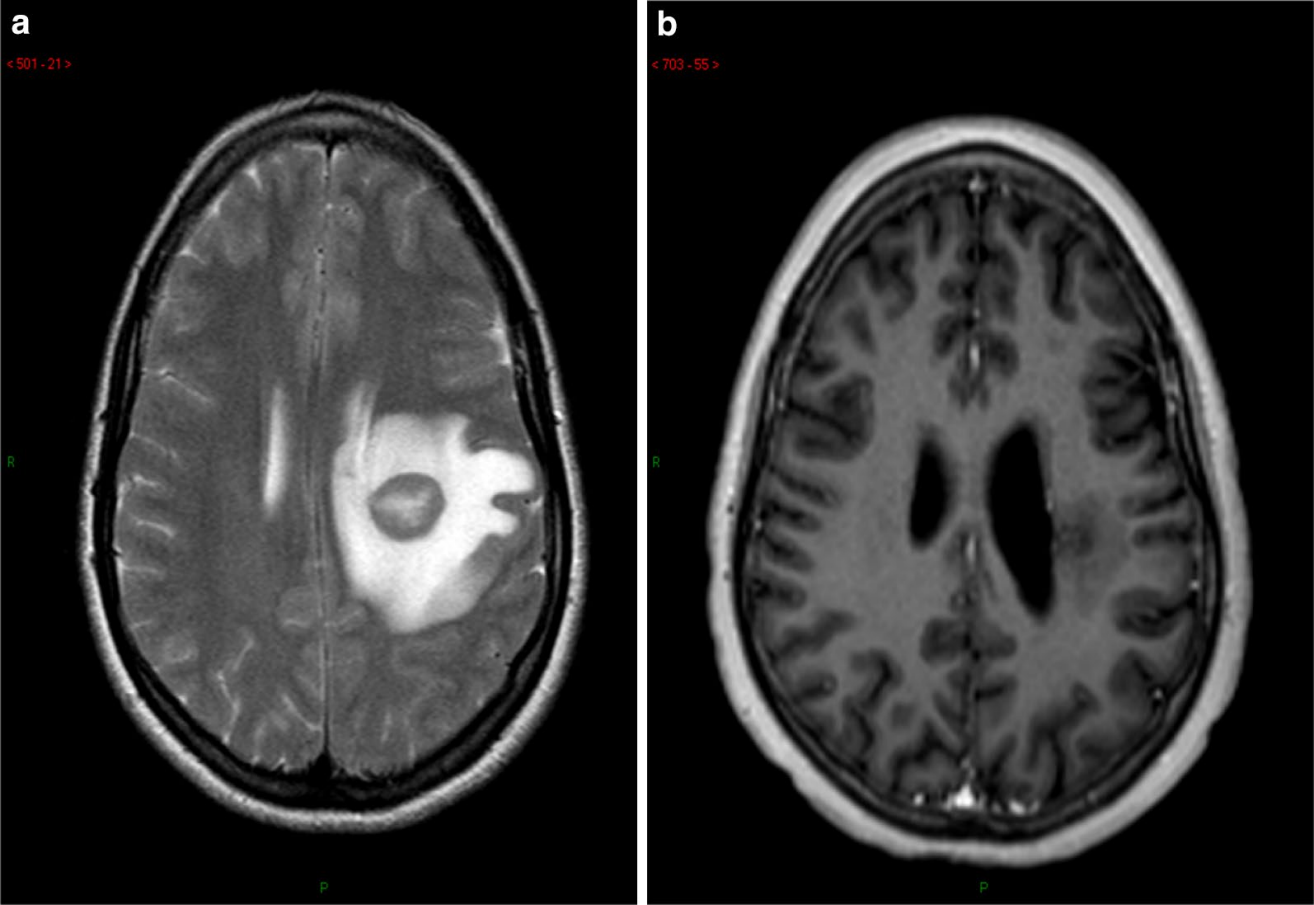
Brain MRI at diagnosis (a) and after two treatments by chemotherapy and radiotherapy (b)
